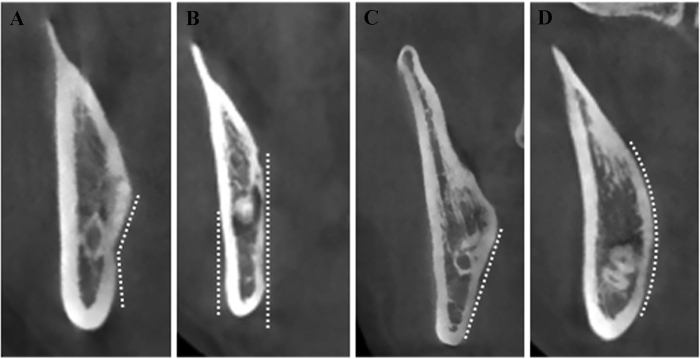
Morphology of lingual plate at the level of root apex identified on cross-sectional CBCT image. (A) Type U, undercut on the lingual side; (B) Type P, parallel to the buccal plate; (C) Type S, slanted with buccolingual width reduced on the lingual side; (D) Type R, round shape on the lingual side.
